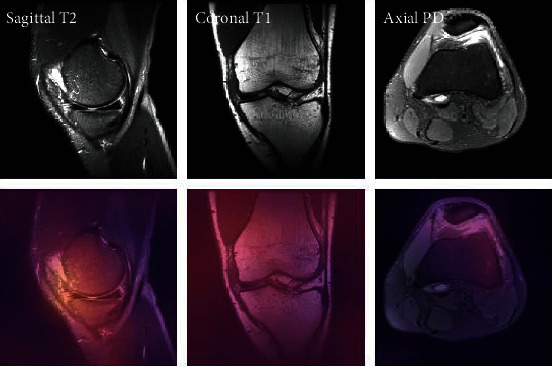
A sample of knee MRI from Osteoarthritis Initiative (OAI) dataset.
